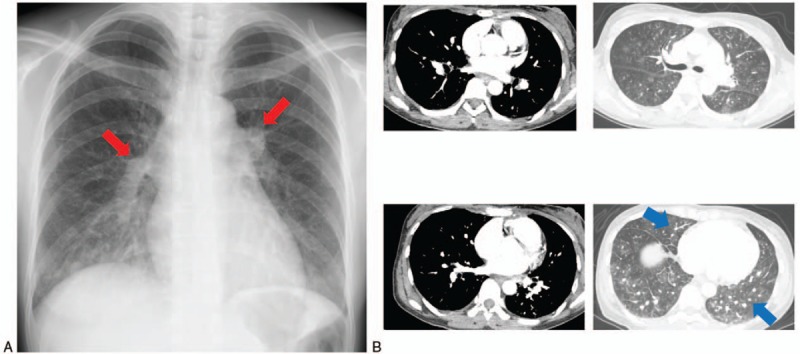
(A) Chest X-ray on admission showed distended pulmonary arteries (red arrowheads) and bilateral interstitial infiltrate. (B) Chest computed tomography on admission showed no pulmonary embolisms in major pulmonary arteries. Nodular opacities with tree-in-bud pattern are seen (blue arrowheads).
